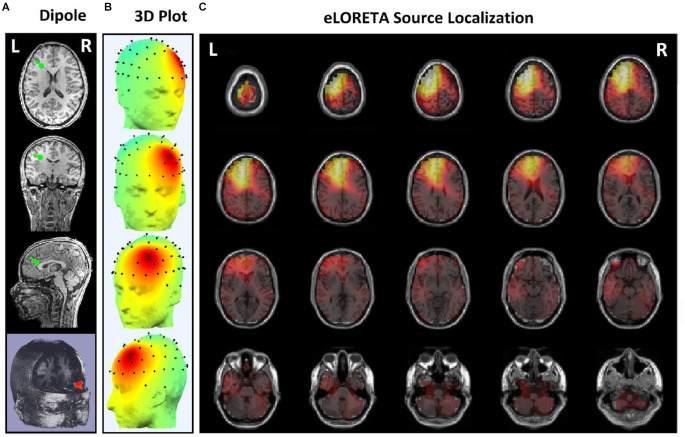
Source localization of rTMS stimulation foci in left prefrontal cortex (L-DLPFC) for a responder subject. (A) Dipole coordinates of the identified focus in left side on a default MRI. (B) Position of foci identified by ICA on the surface of the skull. (C) Source localization in right DLPFC by eLORETA.
